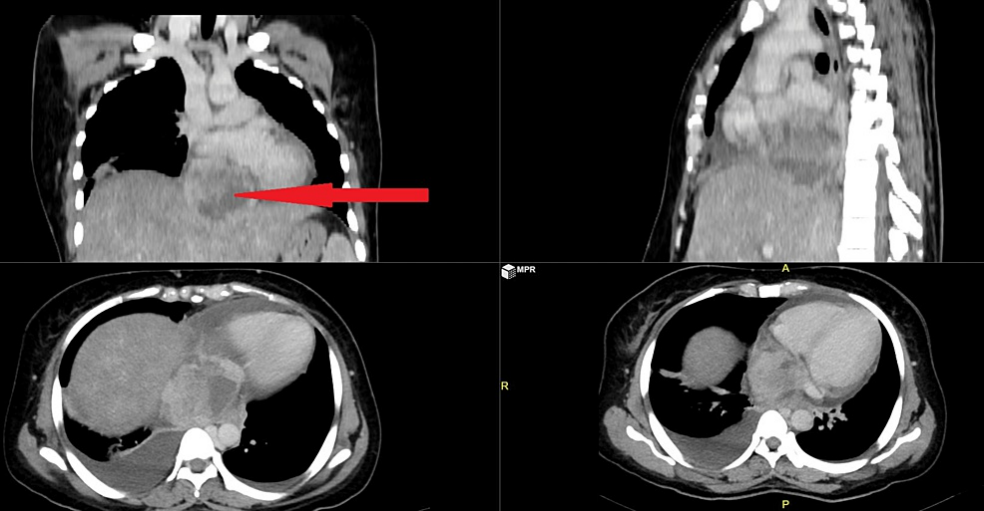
CT abdomen with the red arrow pointing to the supra-hepatic mass
